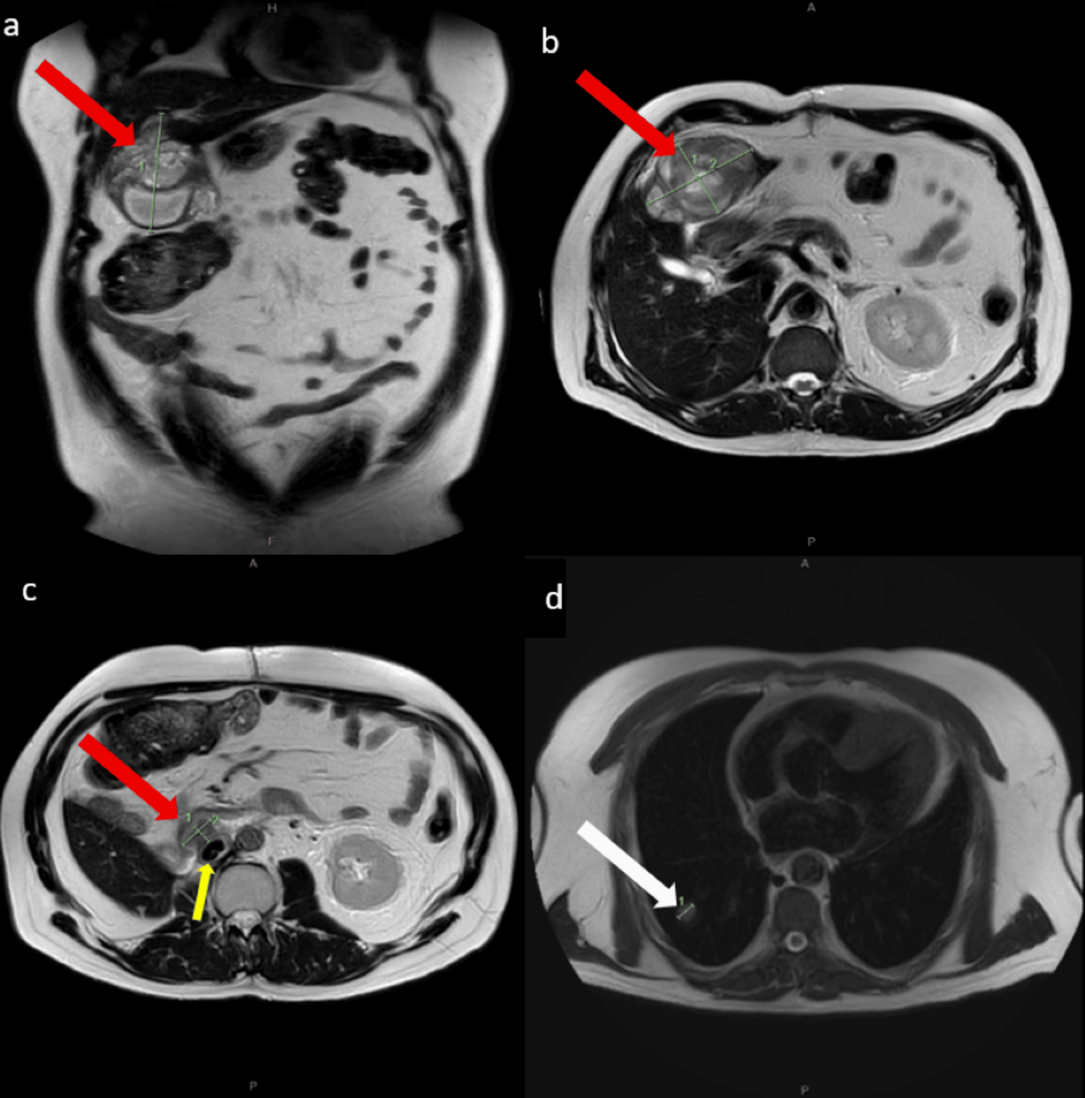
a-d showing MRI T2 phase six months post-operatively a: Coronal slice demonstrating a lesion within the inferior aspect of segment 3 of the liver (red arrow) measuring 8.4 x 5.9 x 9.5 cm (1 = 9.5cm). (b) Axial slice demonstrating a lesion within the inferior aspect of segment 3 of the liver (red arrow). (c) Axial slice showing suspicious recurrence within resection bed (red arrow) intimately related to reconstructed IVC (yellow arrow). (d) Axial slice showing right lung base lesion (white arrow) (1 = 1.36cm)
